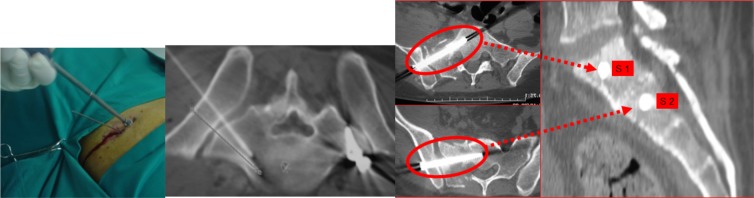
Case of a male patient (46 years old) who sustained a B2 Tile fracture injury with a Dennis III fracture of the iliac wing following a car accident. The first picture from the left shows the positioning of a percutaneous screw. The other three pictures (two coronal and one sagittal view of intra-op CT scan) show the appropriate screw positioning within S1 and S2 vertebral body with preservation of the perisacral neurovascular structures.
